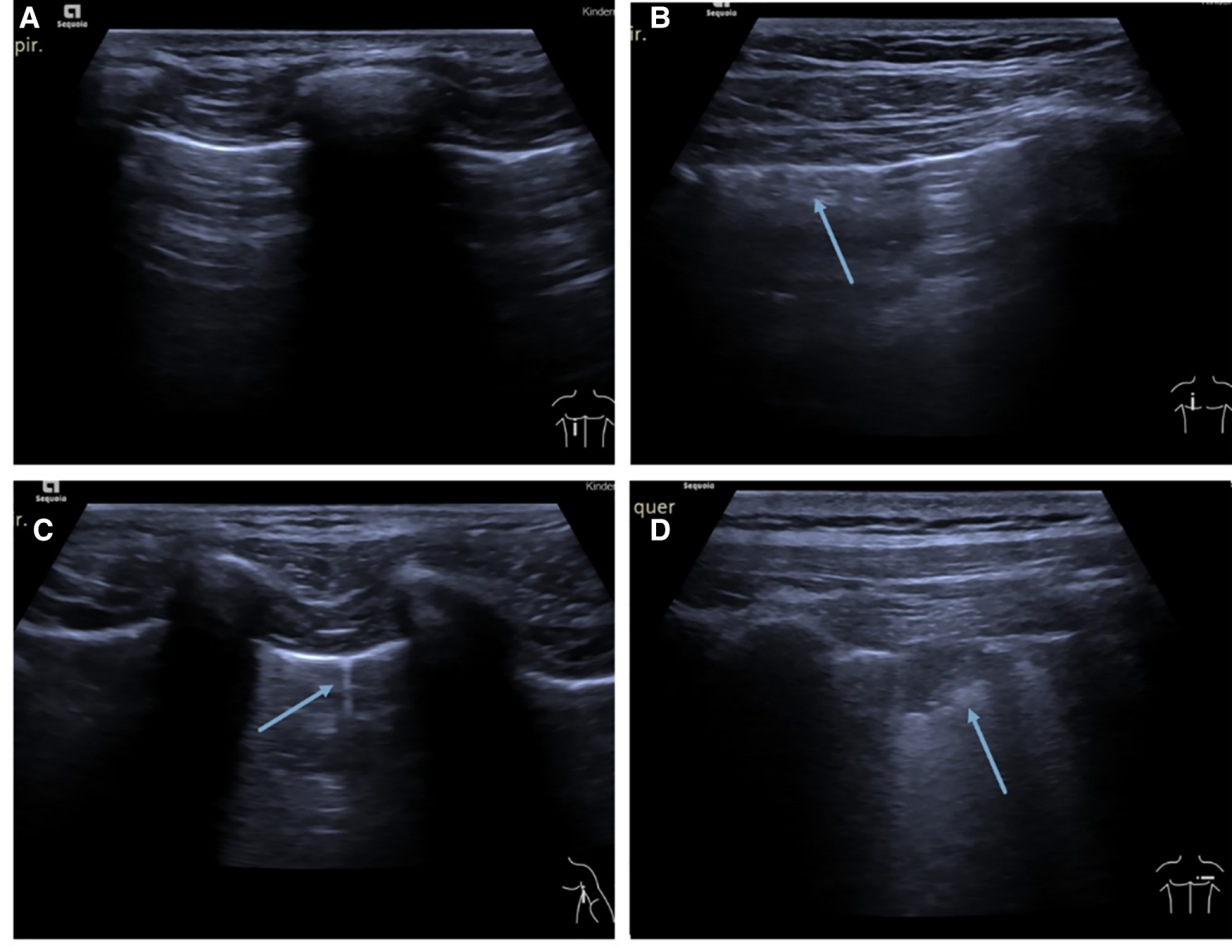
(A) Example of a normal lung ultrasound. Picture showing a typical A-line pattern. (B) Lung ultrasound picture showing pleural irregularity (arrow). (C) Example of a typical B-line (arrow). (D) Example picture of a typical consolidation (arrow).
